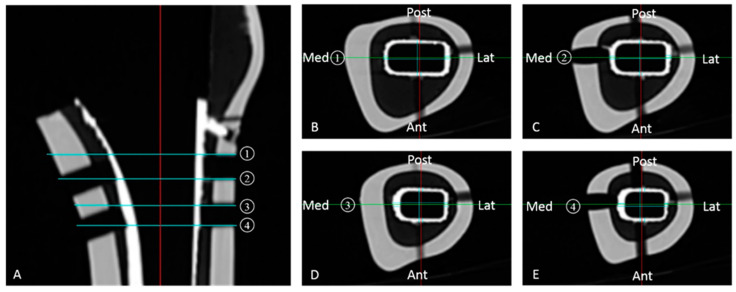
CT images, taken after stem removal. CT images were taken at 1 mm slice spacing in four horizontal sections aligned with the insertion holes of the proximal lateral femoral rods to measure the cement thickness. (A) Coronal slice of femoral canal center. 1: Upper edge of proximal lateral hole for rod. 2: Lower edge of proximal lateral hole for rod. 3: Upper edge of distal lateral hole for rod. 4: Lower edge of distal lateral hole for rod. (B) Axial slice at the level of 1; (C) Axial slice at the level of 2; (D) Axial slice at the level of 3; (E) Axial slice at the level of 4. The thickness of the cement layer was measured at the midpoint of each area.
